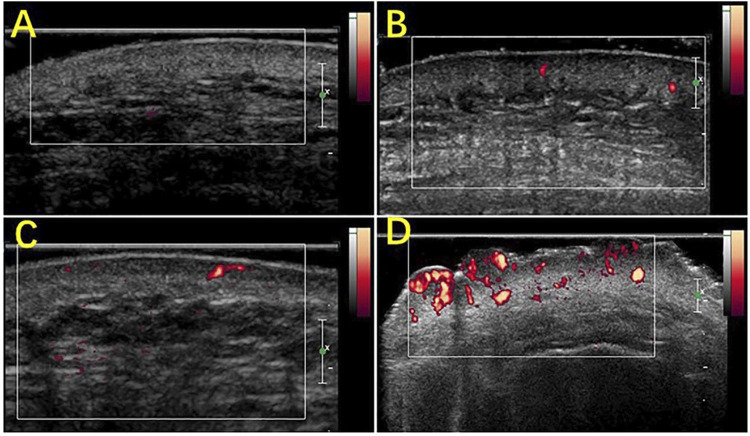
Scores of the blood flow signals in PWS lesions with Power Doppler ultrasound. (A) Grade 0, absence of color signals in a pink-type lesion; (B) grade 1, mild color signals in a thickened-type lesion; (C) grade 2, moderate color signals in a thickened-type lesion; (D) grade 3, marked color signals in a nodular-type lesion.
